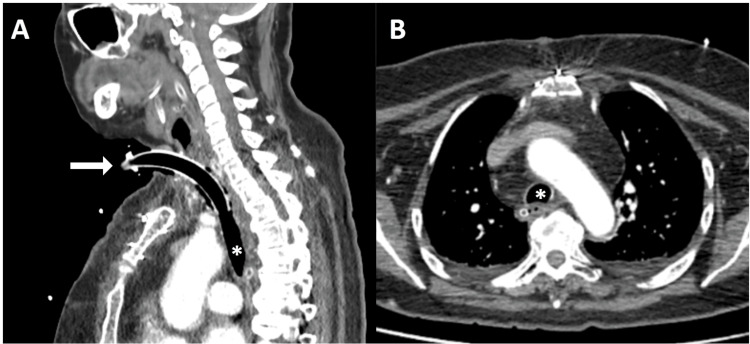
Postoperative CT scan performed after second surgery. Axial (A) and saggital (B) images showed a tracheotomy (arrow) and complete excision of the residual left goiter. The airway was patent with resolution of the tracheal compression (asterisks).
